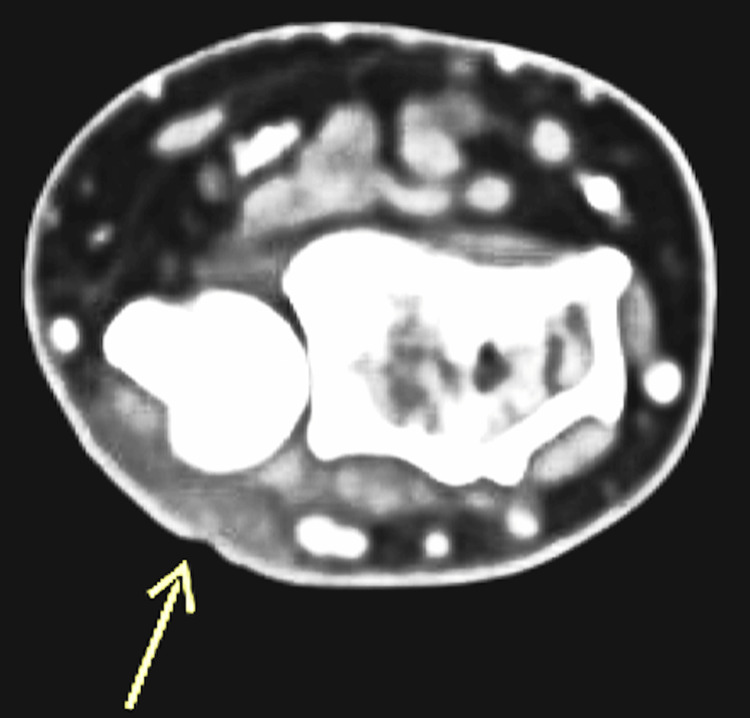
CT scan of upper extremity, sagittal view The arrow points to the fat stranding, which is concerning for soft tissue infection without osteomyelitis.
